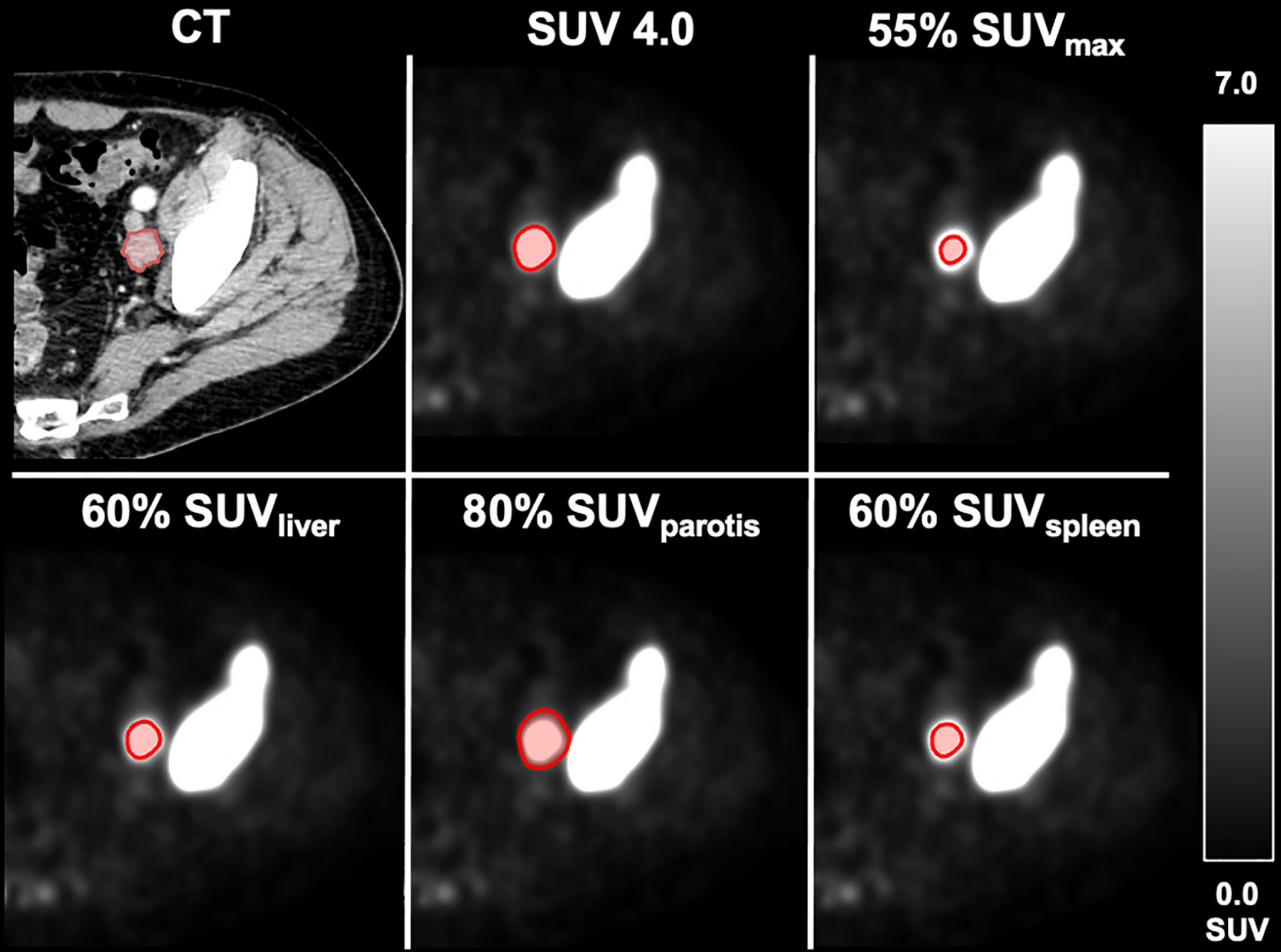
Different delineation methods in an exemplary metastatic patient. Volumetric reference standard 6.3 m; SUV 4.0: 5.5 ml. 55% SUV_max_: 1.0 ml. 60% SUV_liver_: 4.5 ml. 80% SUV_parotis_: 6.4 ml. 60% SUV_spleen_: 4.0 ml.
